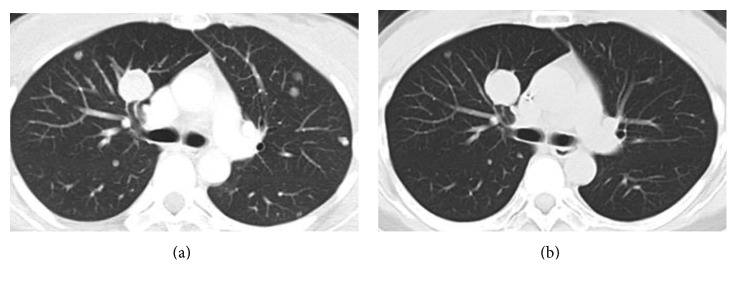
Computed tomography findings. (a) After four courses of IFM/DXR. (b) After 31 months of pazopanib administration. It was classified as no change by the RECIST criteria; however, slight tumor reduction was observed after 31 months of pazopanib administration.
